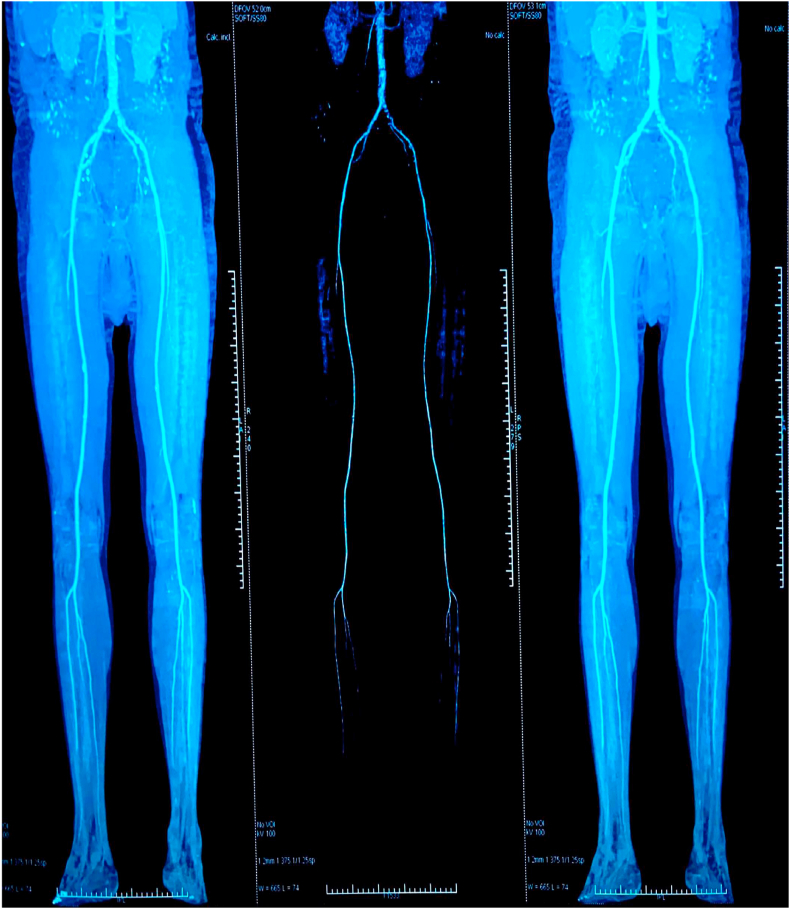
Pre-operative computed tomography angiography with 3-Dimensional reconstruction showing the presence of intraluminal thrombus at iliofemoral artery, total occlusion of popliteal segment, and the absence of flow below.
